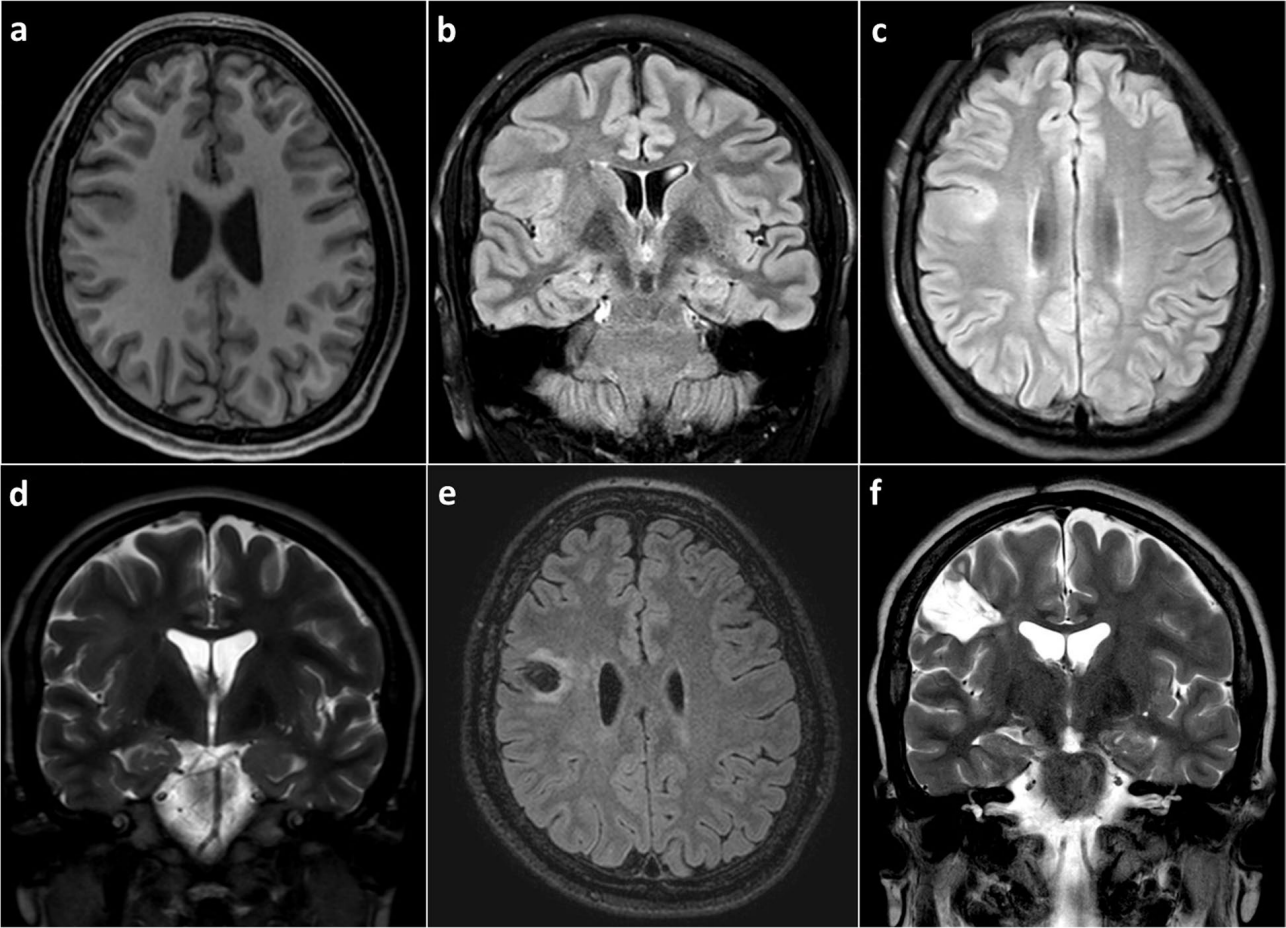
Brain MRI showed cortical thickening and loss of corticosubcortical differentiation in the premotor area on axial T1 (a), coronal T2-FLAIR (b), axial T2-FLAIR (c), and coronal T2 (d) images. Brain MRI performed after the procedure showed complete resection of the lesion on axial T2-FLAIR (e) and coronal T2-weighted (f) images
